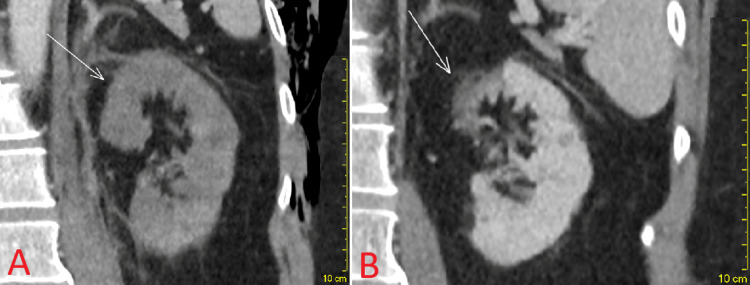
Appearances on the left kidney on the initial CT (panel A) and follow-up 11 months post-discharge (panel B). Note that the maximum cortical thickness in the upper pole decreased from 1.7 to 1.0 cm (arrows).
